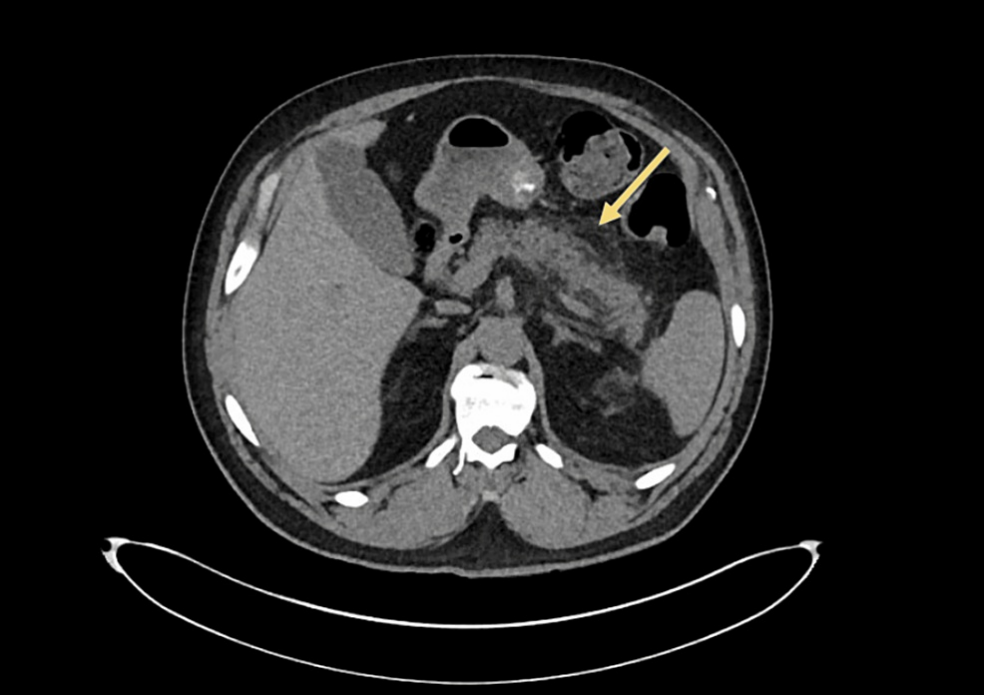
CT abdomen/pelvis w/o contrast showing extensive interstitial edema around the pancreatic tail along with peripancreatic fat stranding
